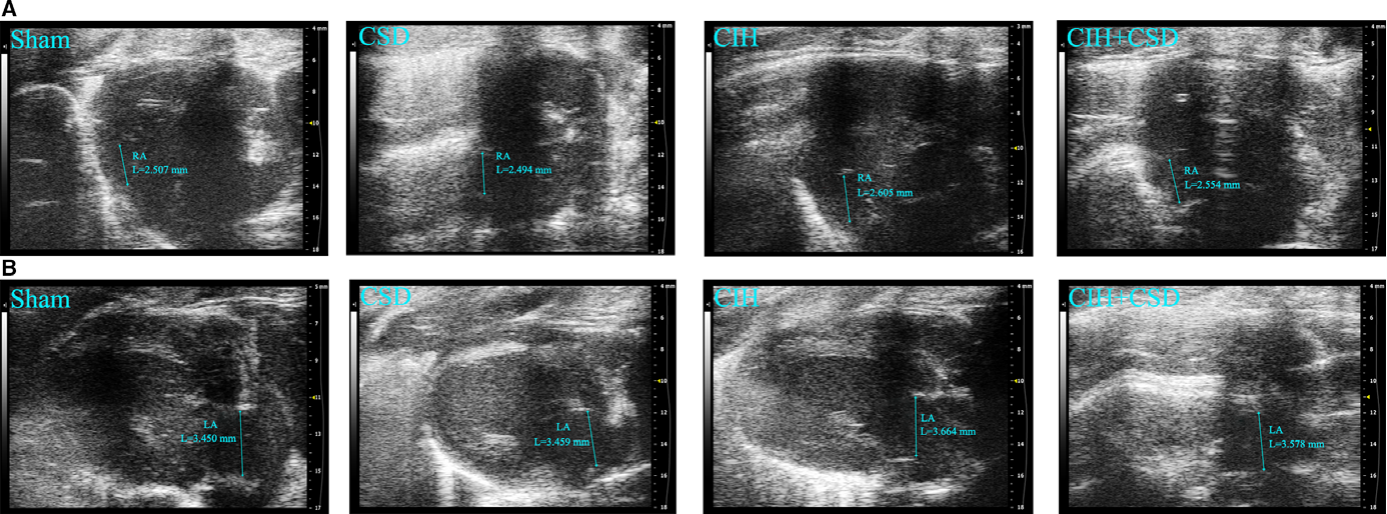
Ultrasonography shows the images of the RAD and LAD. A, CIH produced statistically significant RAD dilation, and CSD treatment significantly decreased the RAD dilation. B, LAD was significantly increased after CIH, and LAD was reduced by CSD treatment. CIH indicates chronic intermittent hypoxia; CIH+CSD, chronic intermittent hypoxia with cardiac sympathetic denervation group; CSD, cardiac sympathetic denervation; LAD, left atrial diameter; RAD, right atrial diameter; Sham, sham group.
